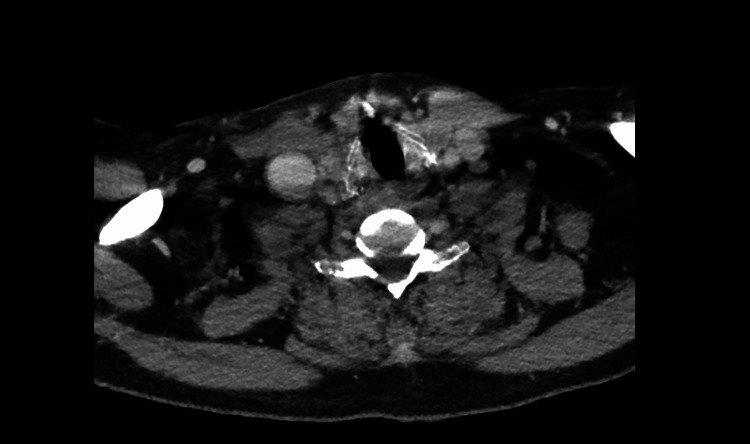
CT scan of the neck showing soft tissue edema surrounding the sternocleidomastoid muscle
